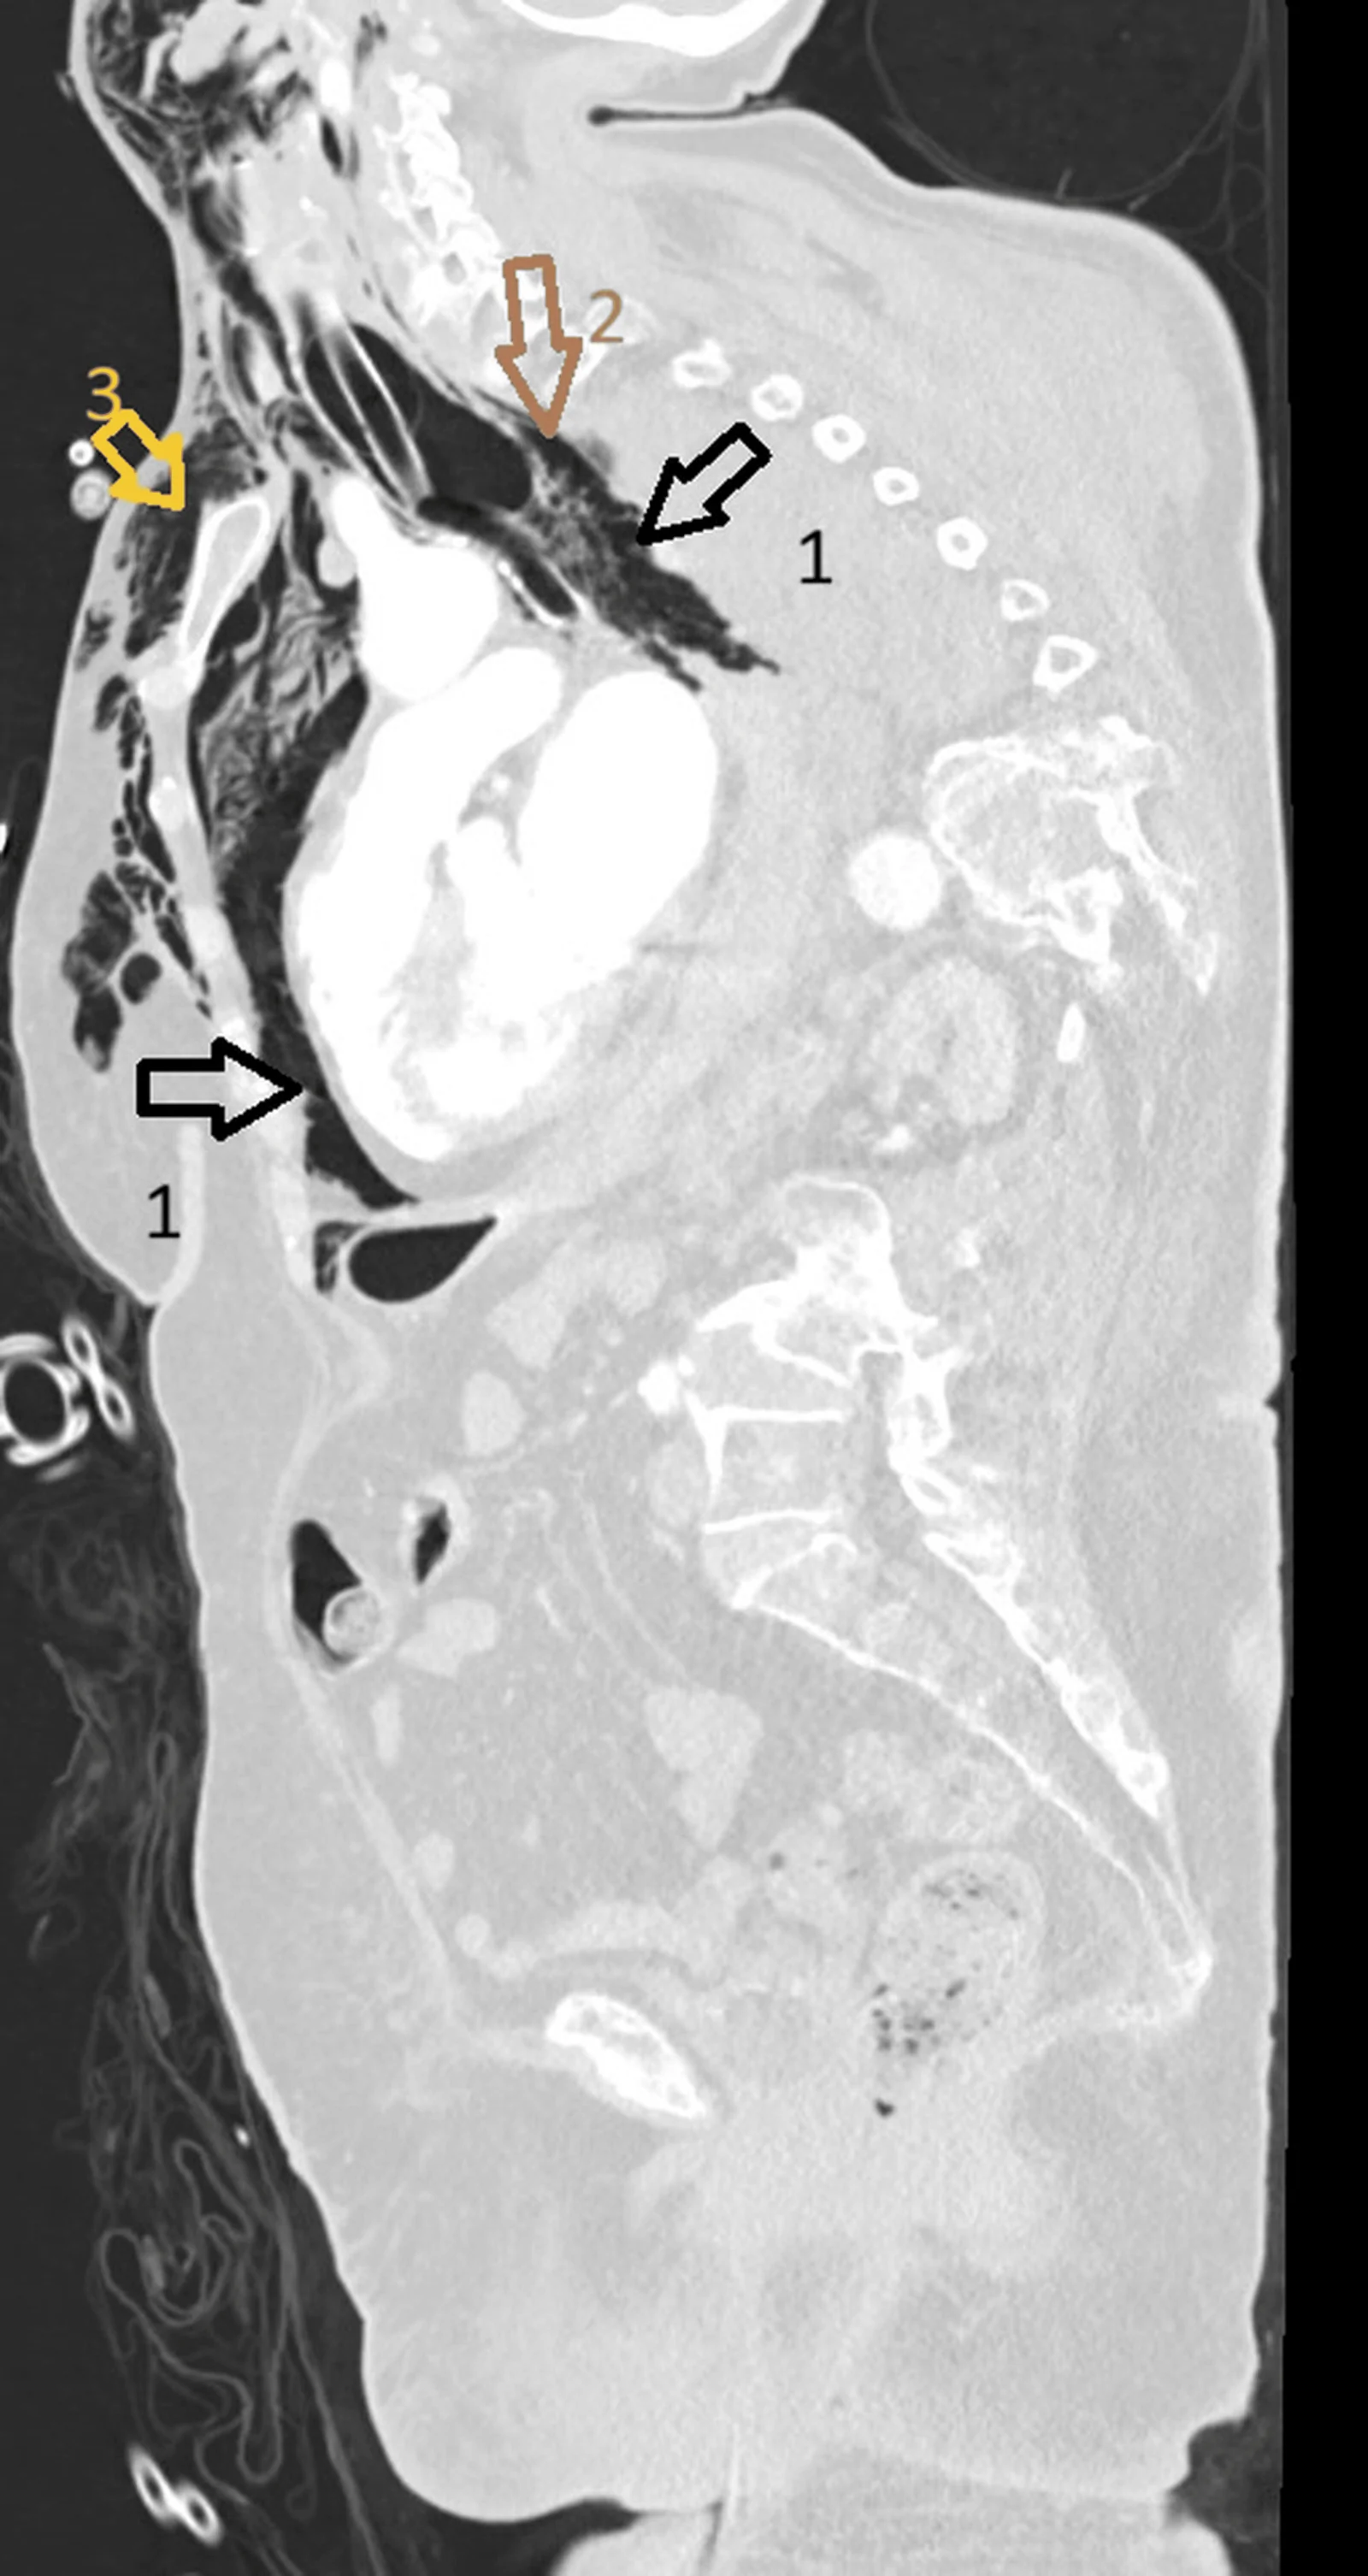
Contrast-enhanced thoracic computed tomography, sagittal reformat Diffuse pneumomediastinum (arrow 1), discontinuity of the posterior membranous tracheal wall (arrow 2) and subcutaneous emphysema of the anterior cervicothoracic soft tissues (arrow 3). The discontinuity corresponds to the lesion identified at bronchoscopy; the full extent found at thoracotomy was not appreciable on this imaging.
